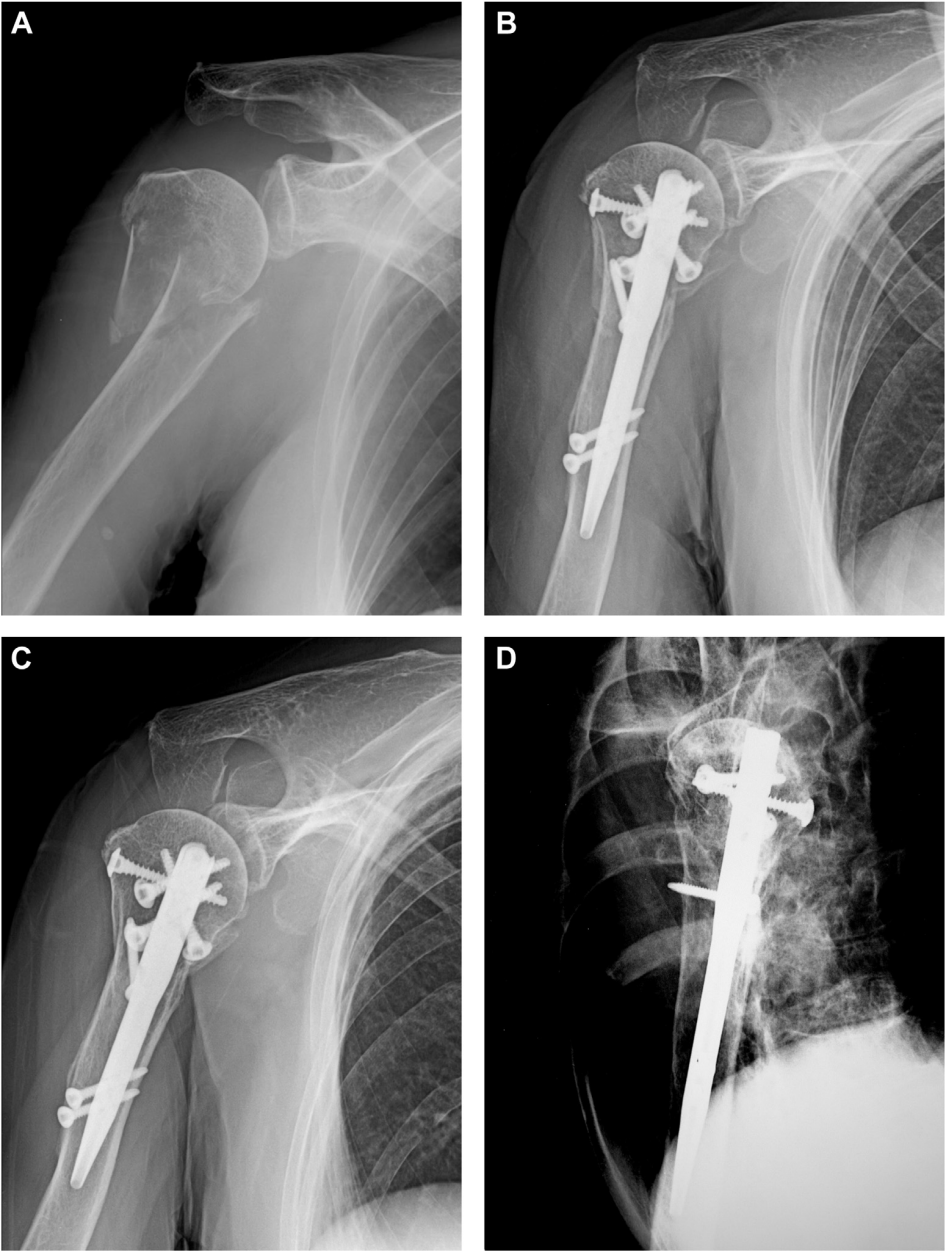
Right* humeral surgical neck fracture of an 88-year-old woman. (A) Anteroposterior radiograph at the time of injury showing medial displacement of the humeral shaft and a wedge fragment in the metaphysis. (B) Anteroposterior radiograph 2 weeks after osteosynthesis. Intramedullary nailing with blocking screw augmentation reduces medial translation, and the neck-shaft angle is 140°. (C) Anteroposterior radiograph at the final follow-up 15 months after osteosynthesis. Bone union is achieved without any correction loss. (D) Scapular-Y radiograph at the final follow-up. Posterior angulated deformity does not remain.
